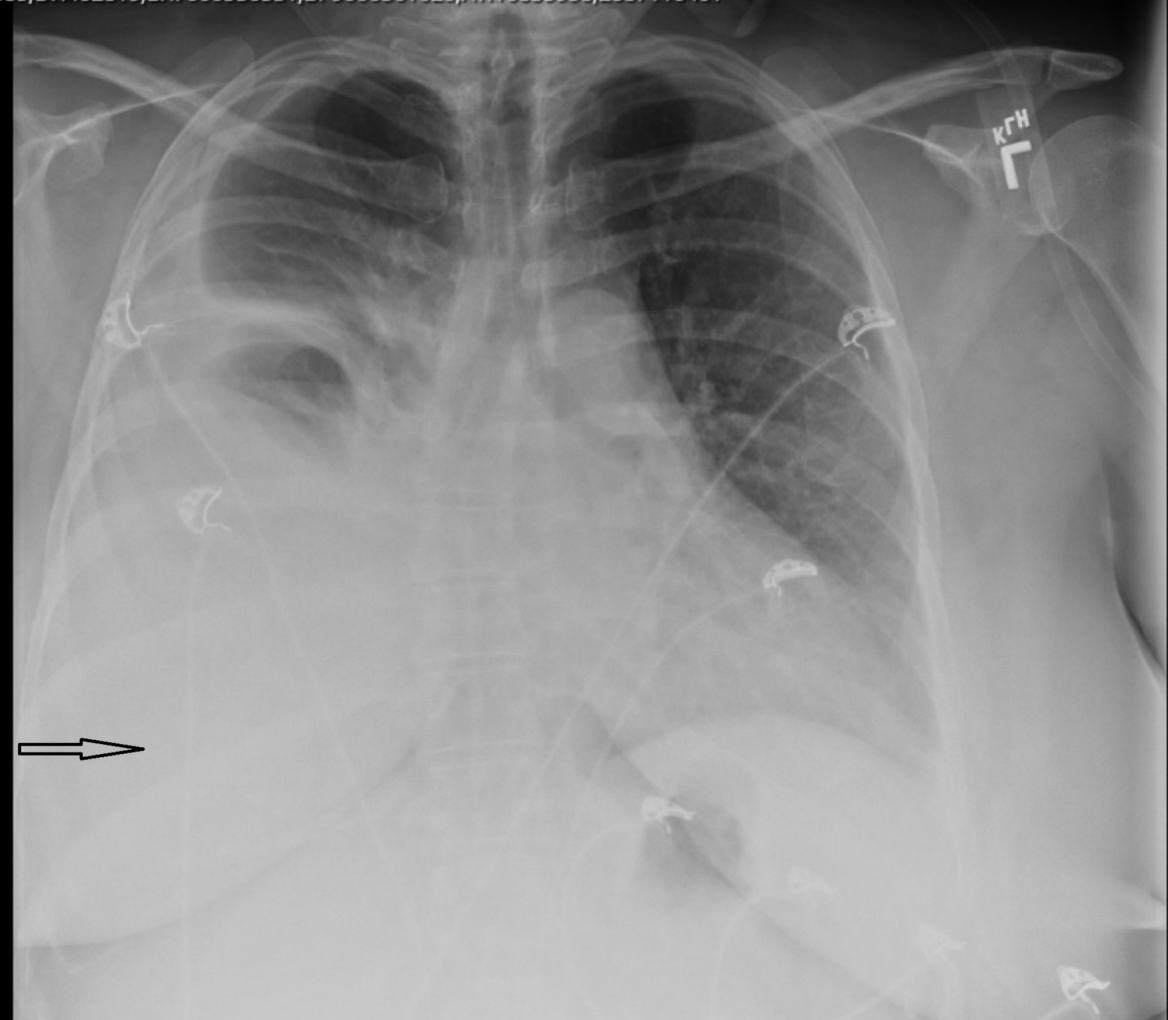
Chest X-ray posterior–anterior (PA) showing a right-sided pleural effusion (arrow)
